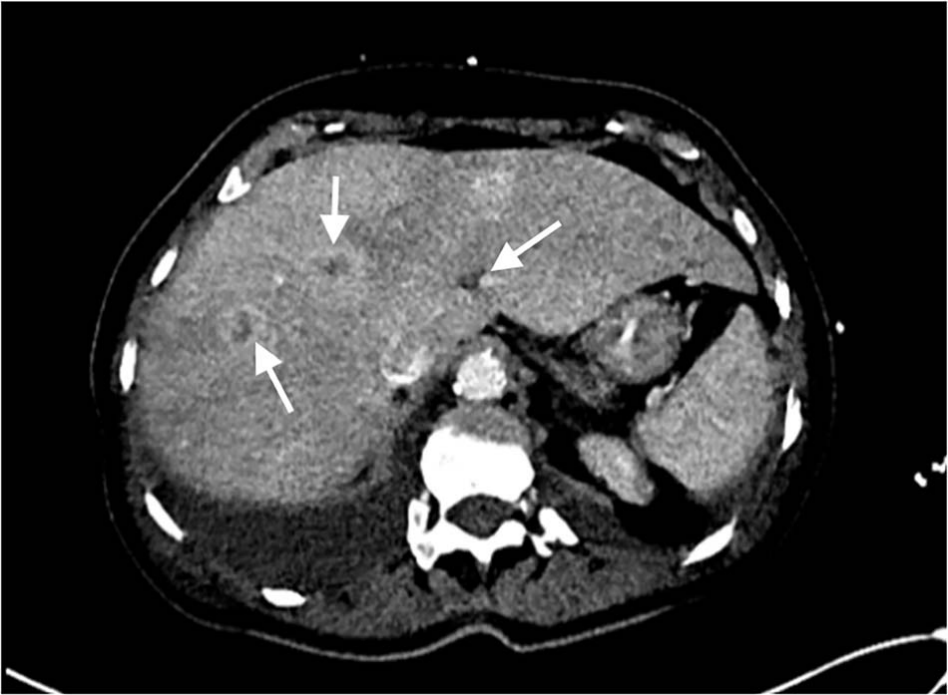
Axial CT image of the liver, showing multiple lesions, initially thought to be septic emboli.
